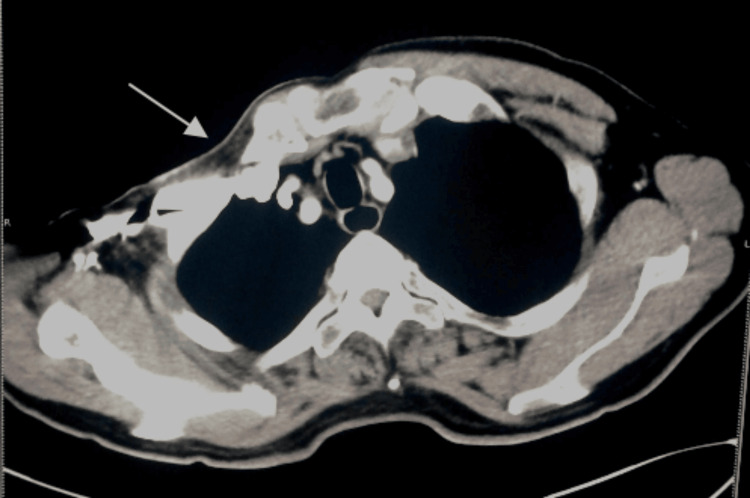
Axial plain CT scan shows pectoral muscles on the left chest but pectoral muscles are absent on the right side (arrows).
